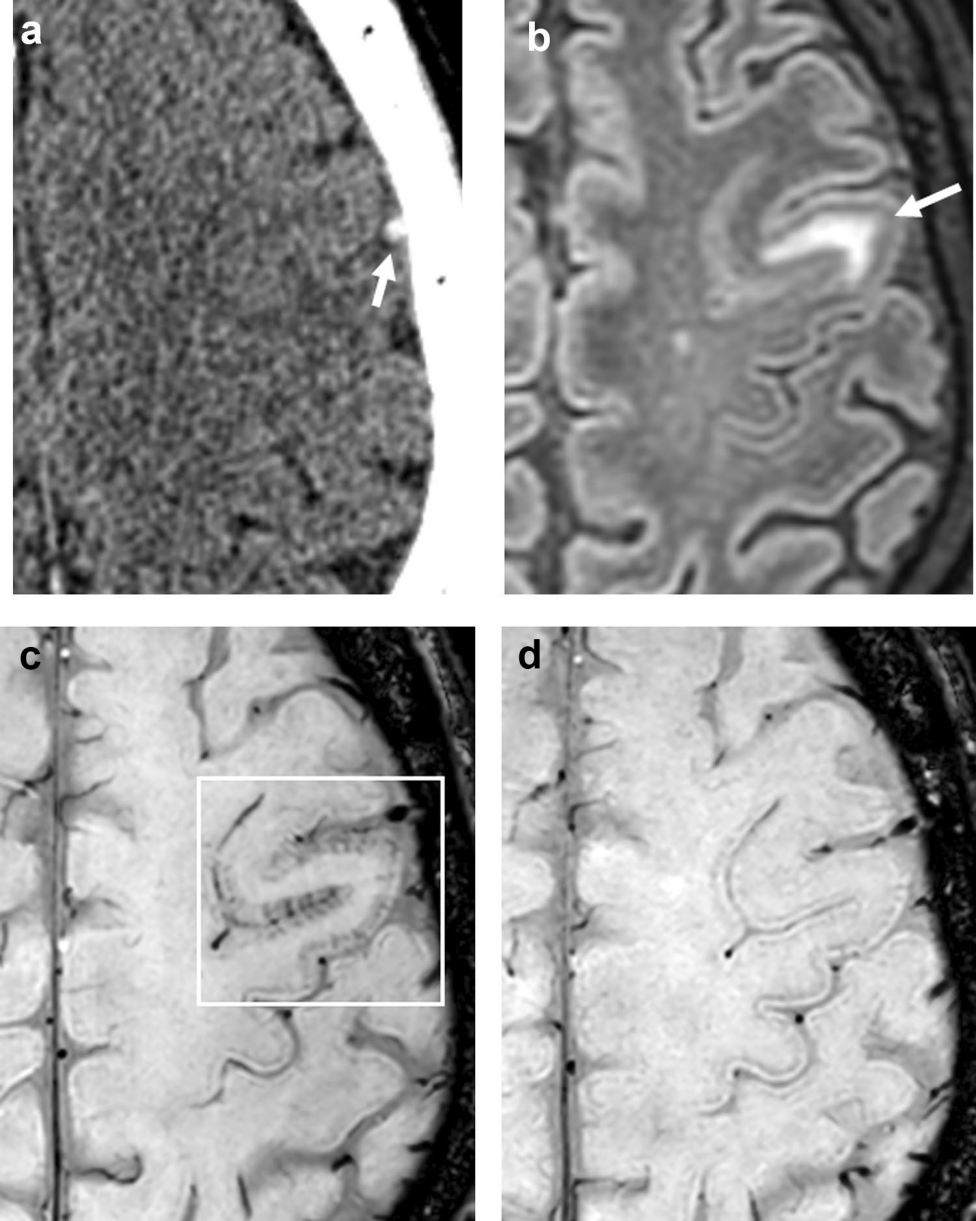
A 60-year-old man presented with headache and seizure. CT and MRI were obtained within 90 min. CT shows a high density of the left cortical vein (a, arrow), indicating cortical vein thrombosis. Contrast-enhanced CT revealed thrombus in superior sagittal sinus but patency of the deep venous system (not shown). FLAIR shows edema in the left gyrus (b, arrow). SWI shows the cortical brush sign in the affected gyrus (c, square). After anticoagulation treatment, SWI shows disappearance of the cortical brush sign (d)
